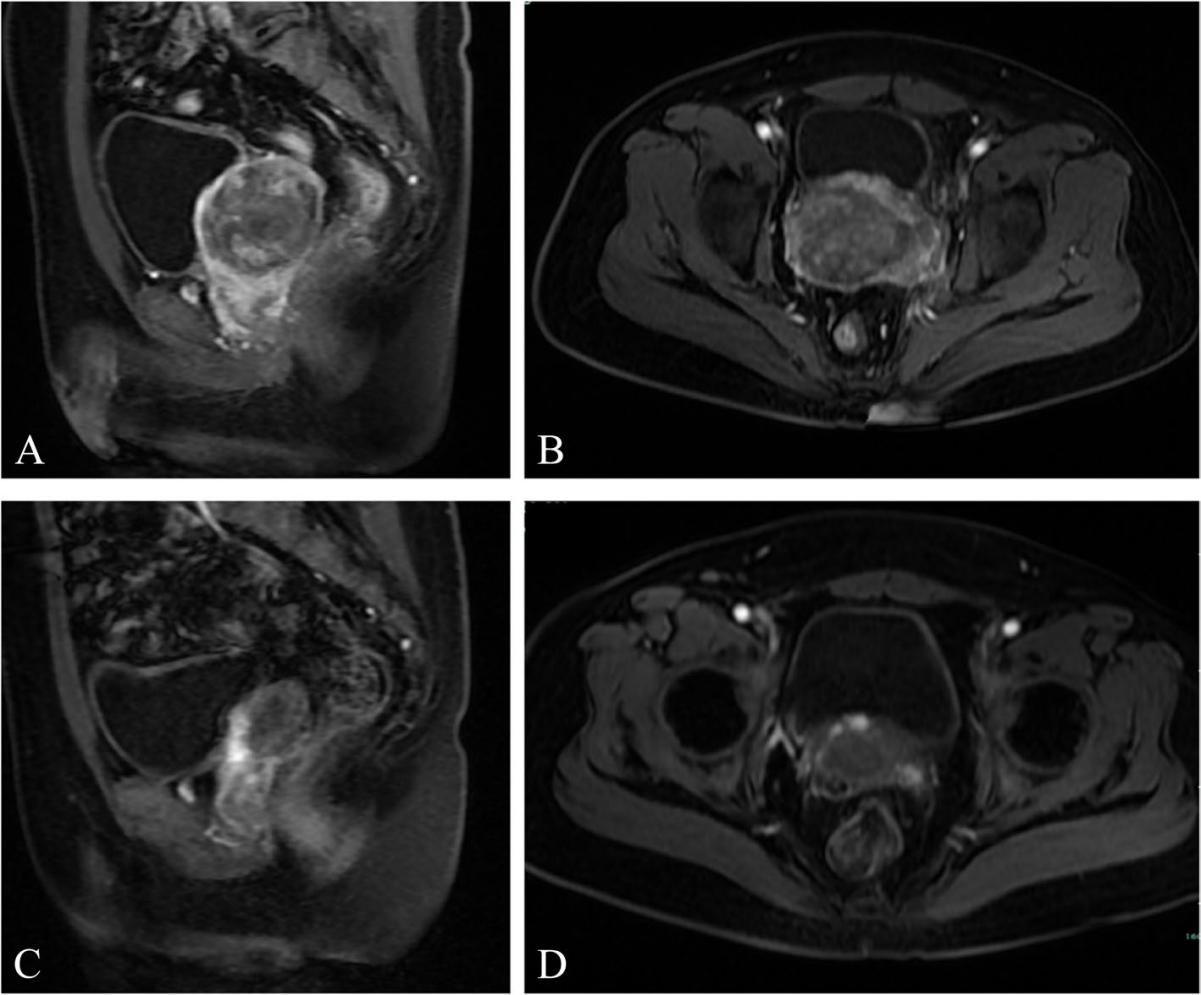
Prostate MRI images A In the pre-chemotherapy images, the sagittal T2-weighted images (T2WI) indicated the presence of a mass in the middle and upper regions of the prostate. The mass showd mixed signals and had unclear boundaries. B In the T1-weighted imaging (T1WI) sequence, fat inhibition revealed clear and uneven enhancement surrounding the tumor, without any internal enhancement. C, D After chemotherapy treatment, the size of the mass and the lymph nodes in the left iliac vessel noticeably decreased
